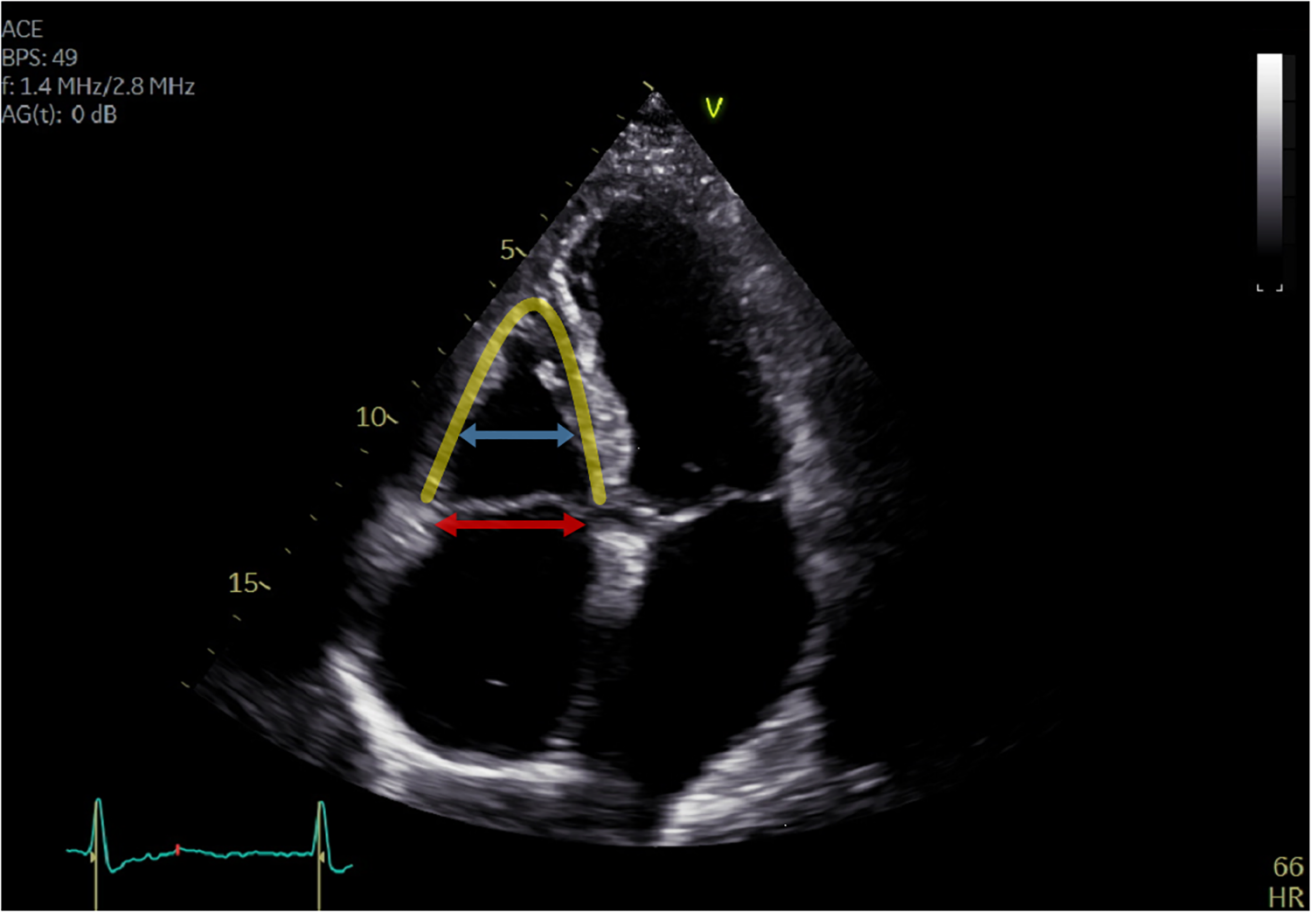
Apical four-chamber view of a patient with atrial functional tricuspid regurgitation. Tricuspid regurgitation is mainly caused by right atrial enlargement with consequent tricuspid annulus dilatation (red arrow). The right ventricle is triangular shaped (yellow line) without remodelling or dilatation (blue arrow), and the coaptation line is found at the height of the tricuspid annulus (<10 mm tenting height).
